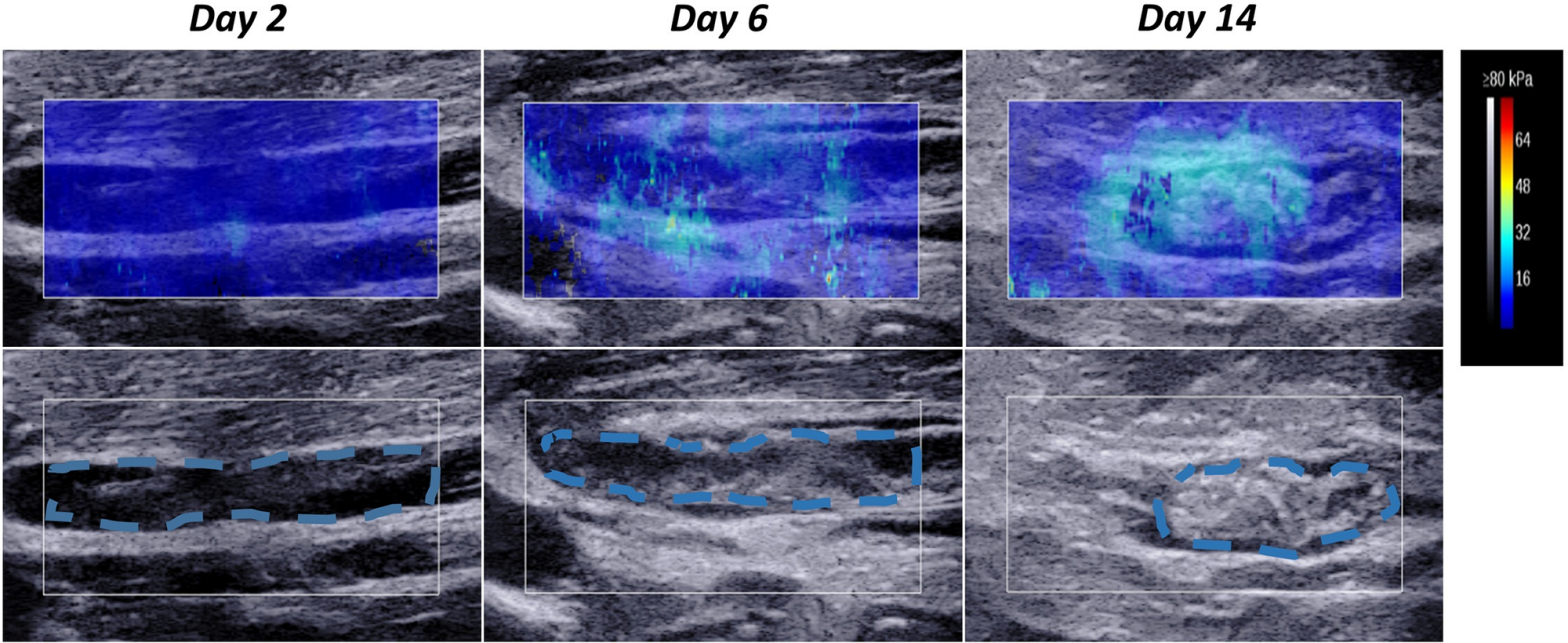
Ultrasound variation of the ROI in the thrombus of rabbit #35 on day 2 (left), day 6 (middle) and day 14 (right). The lower row shows gray-scale ultrasound changes in the ROI, the thrombi in the ROI are traced by the blue dashed line; the upper panel shows the shear wave elastography (SWE) changes in the ROI. The vein under analysis and the adjacent tissue are indicated by a solid-line rectangular frame. Thrombus hardening correlates with a gradual color change from dark blue to deep red in the SWE images. The Young's modulus (YM) values were 3.15 kPa (day 2), 7.20 kPa (day 6) and 12.39 kPa (day 14).
